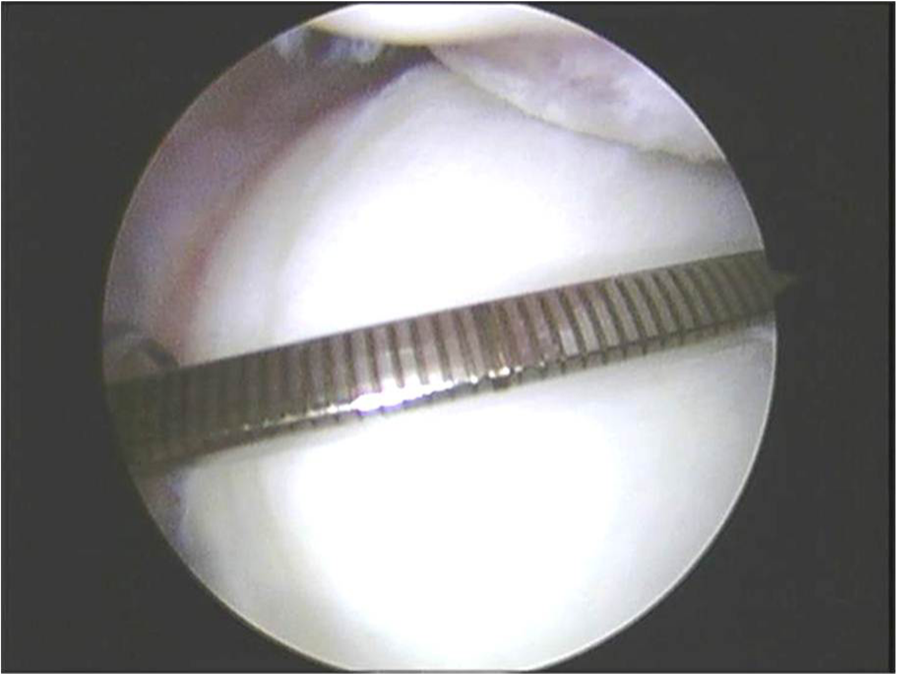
Computed tomography showing the glenoid with the measurements of each segment.
